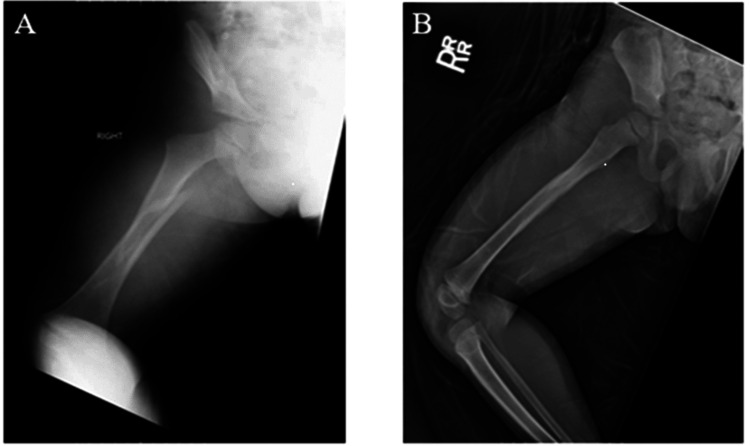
Initial imaging for case 4, including (A) AP and (B) lateral radiographs of the right lower extremity demonstrating a spiral fracture of the femoral shaft
